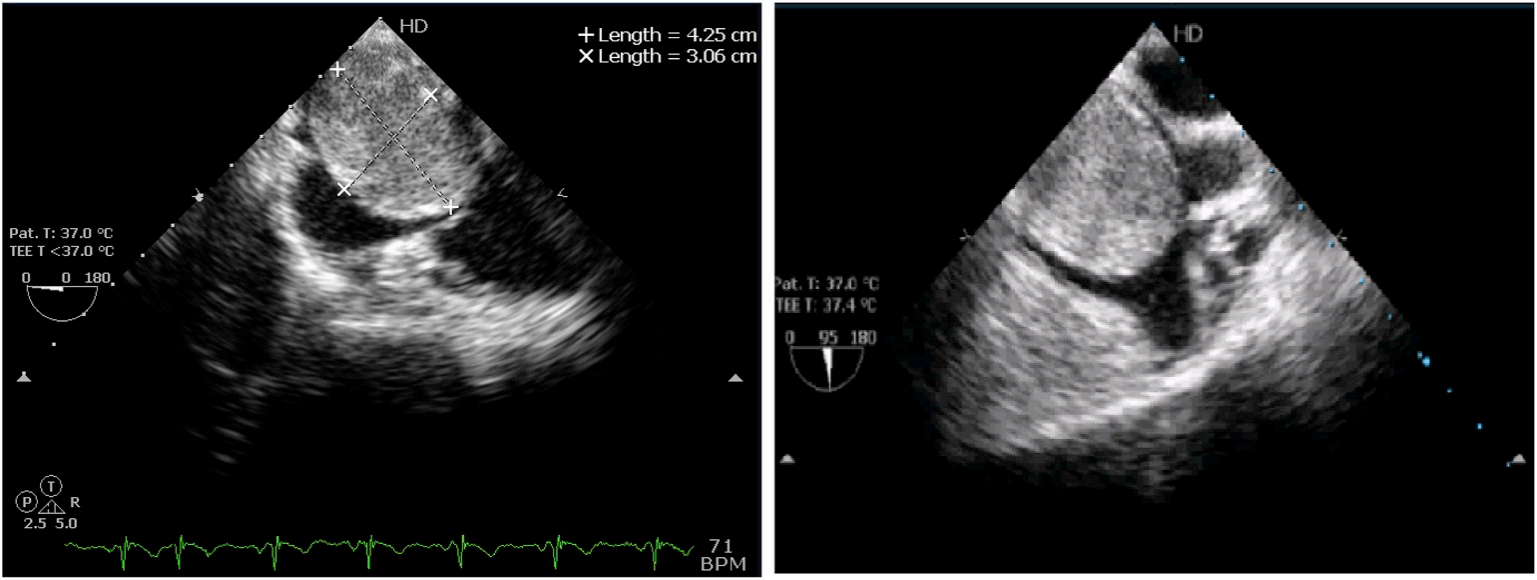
2D Echocardiography showing a large mass in the right atrium.
